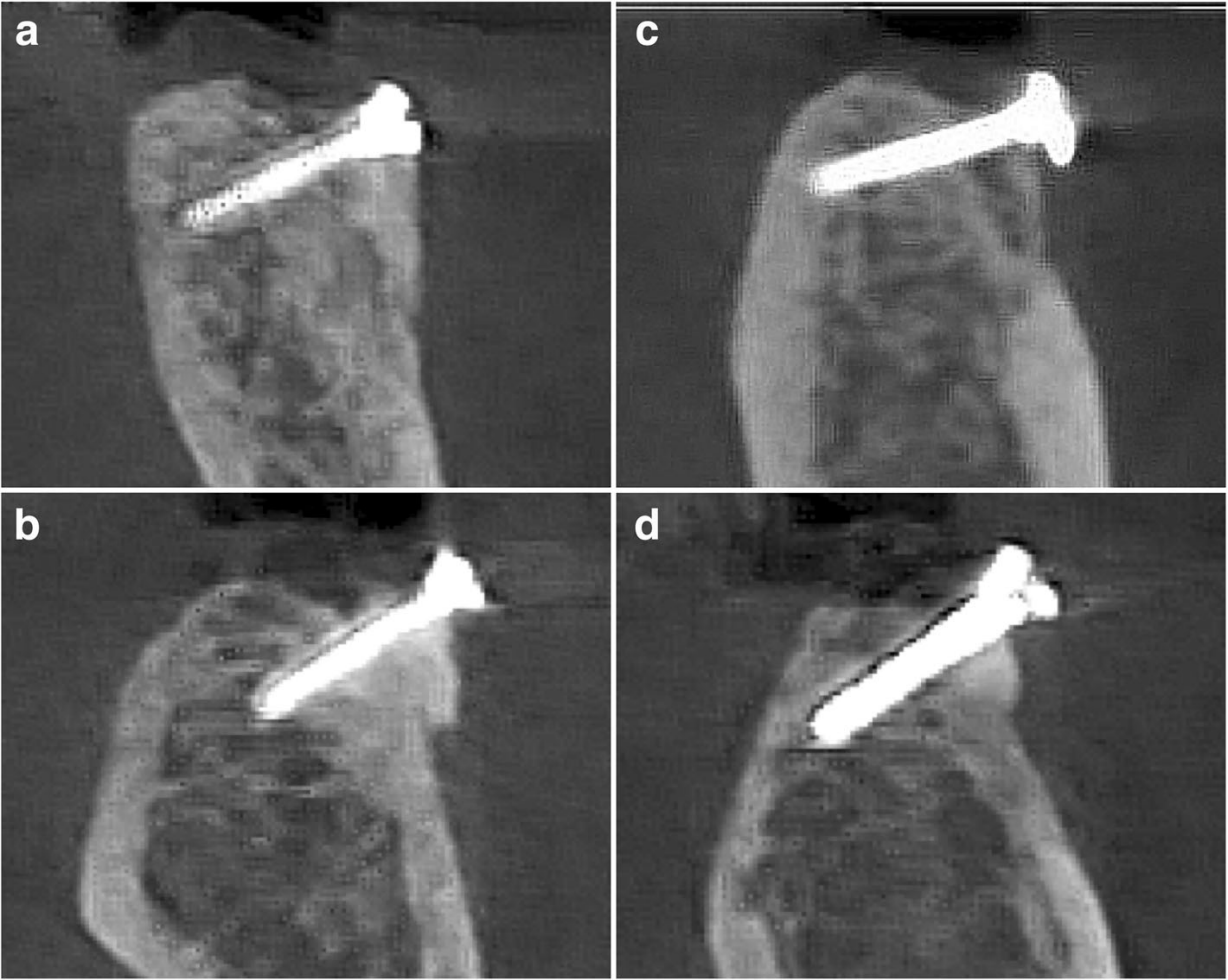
Representative CBCT outcomes at 26 weeks. a, b TR graft. c, d AB graft
